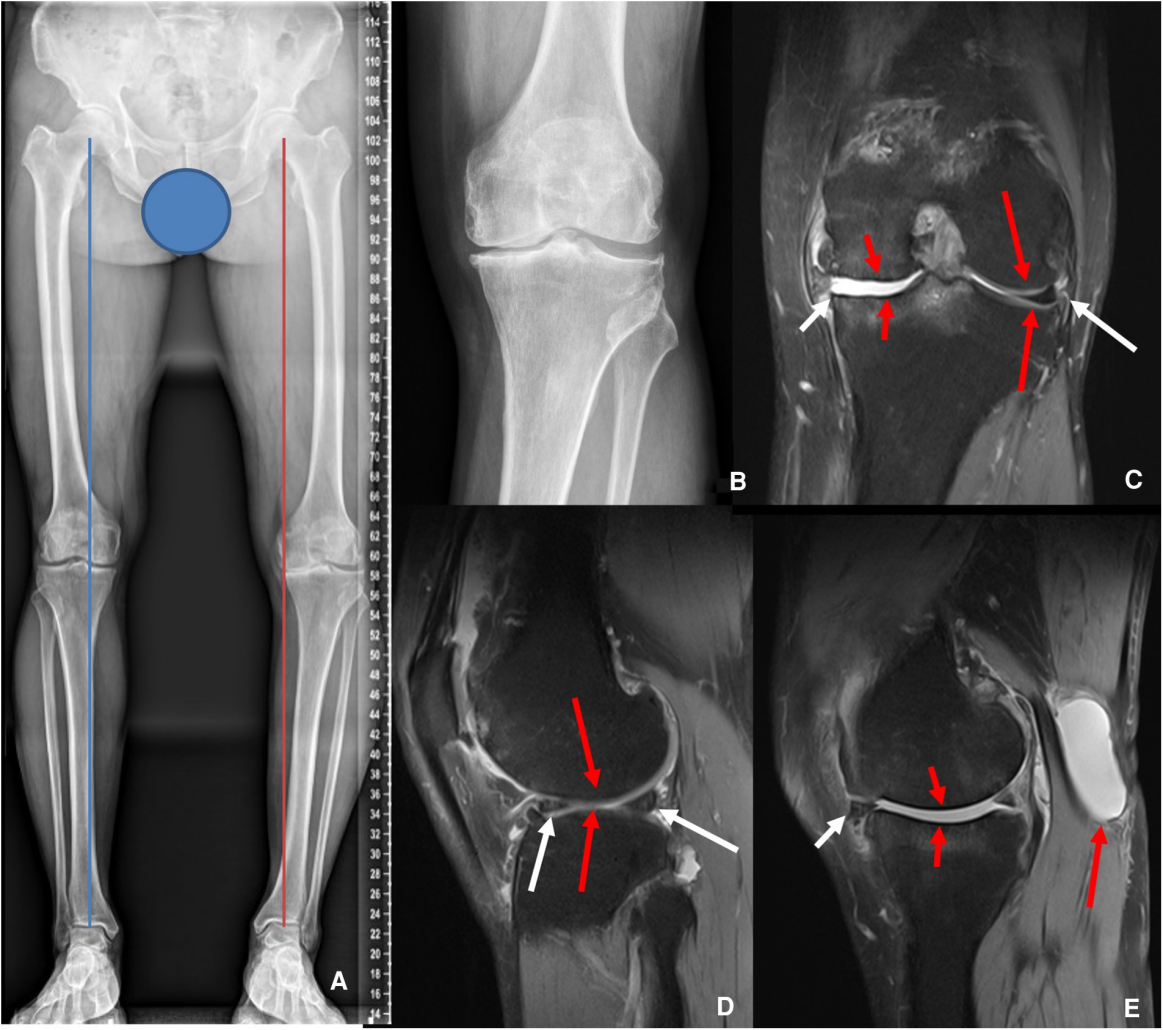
Preoperative X-ray and MRI. (A) The left Weight-Bearing line (red) was 35 mm more varus than the contralateral side (blue). (B) Narrowing of the medial compartment of the left knee. (C,D) The short white arrow showed subluxation of the medial meniscus. The short red arrows showed the cartilage wearing on both sides of the medial femur and tibia. The long red arrows showed the normal cartilage on the lateral side of the knee. The long white arrows showed the lateral meniscus was intact. (E) The short white arrow showed subluxation of the medial meniscus. The short red arrows showed the cartilage wearing. The long red arrow indicated the popliteal cyst.
